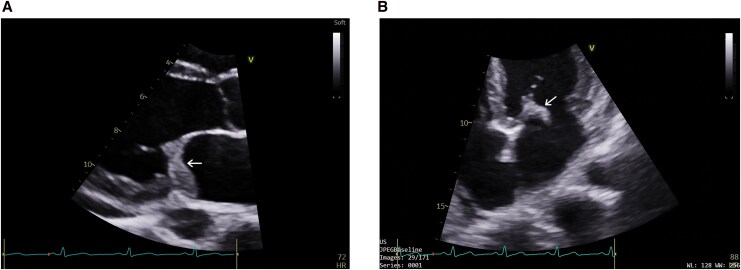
2D transthoracic echocardiography on presentation. (*A*) (Parasternal long axis view), (*B*) (A4C-Focused mitral valve) shows a large, mobile echogenic mass attached to the anterior leaflet of the mitral valve (marked by white arrow).
